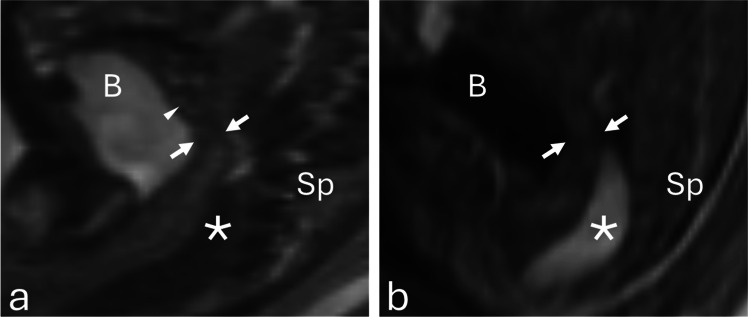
a Sagittal T2-weighted MR image shows the uterus posterior to the urinary bladder (B), with hypointense myometrium (arrows) and mildly hyperintense endometrium (arrowhead). b Sagittal T1-weighted MR image shows T1-hyperintense meconium within the rectosigmoid colon (asterisk), posterior to the uterus (arrows). Measurements were made from the outer margin to the outer margin across the anteroposterior dimension of the endometrium
